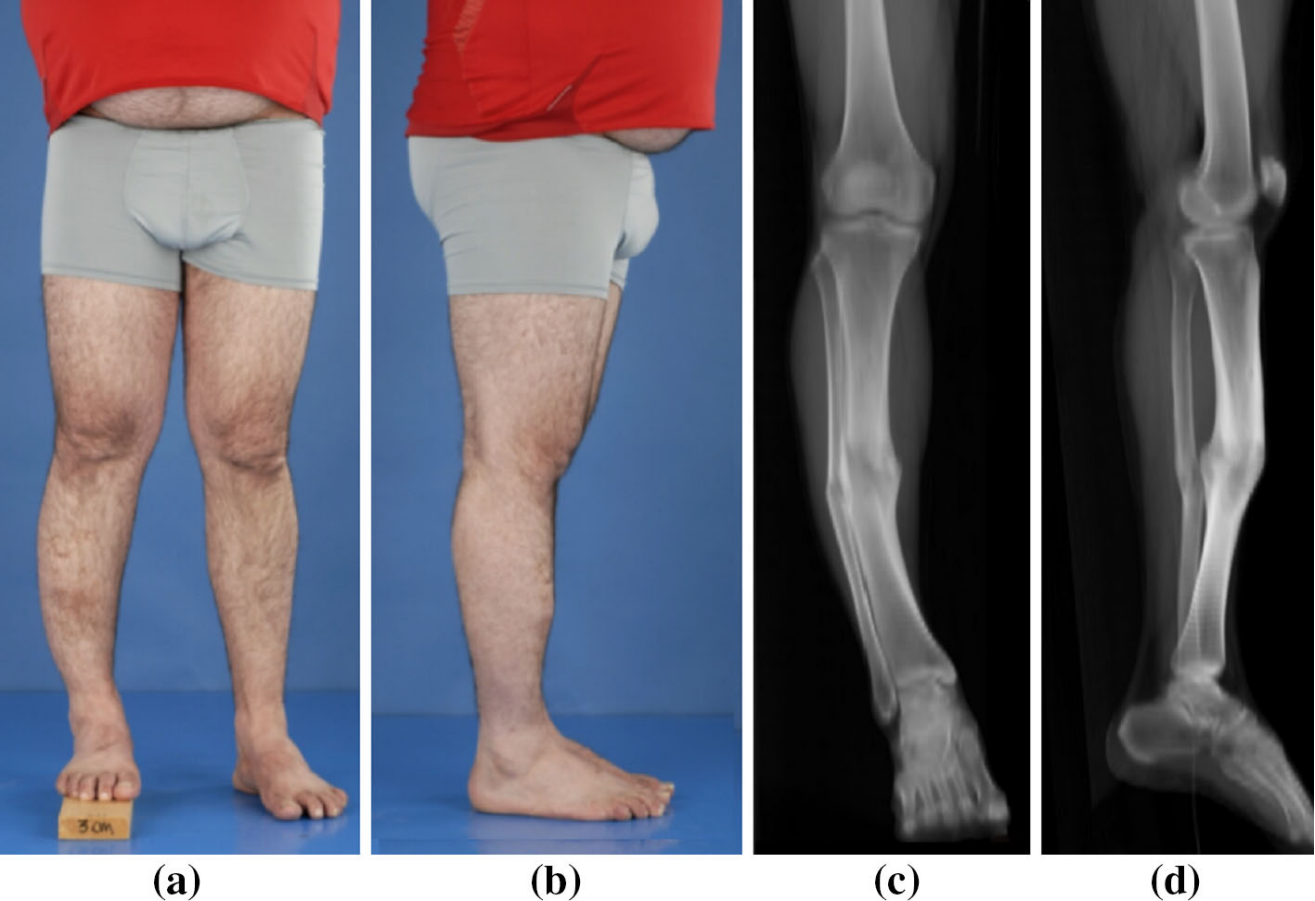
Preoperative malunion deformity of the right lower leg. a Frontal and b lateral photographic images. Digitally reconstructed radiographic images in c AP and d lateral view
